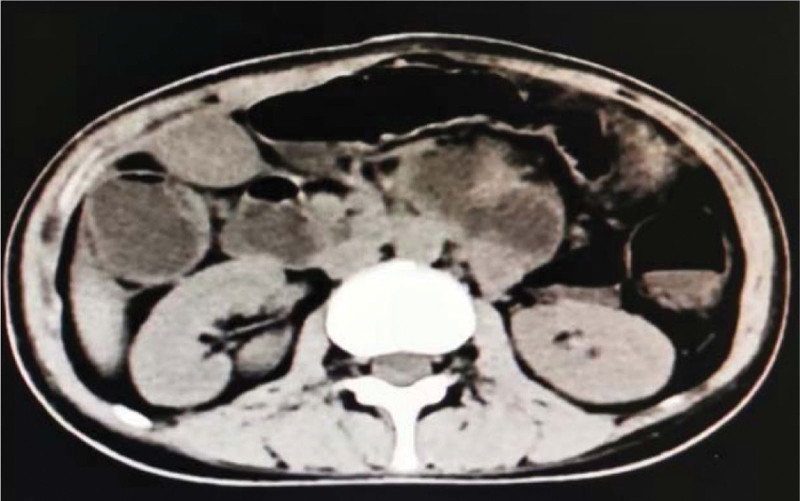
No liquid pneumoperitoneum sign in abdominal CT.
